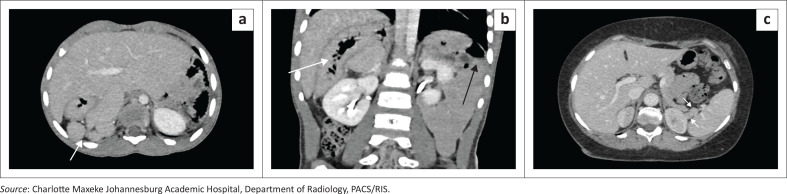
Heterotaxy syndrome identified incidentally in a 6-year-old trauma patient. (a, b) Axial and coronal contrast-enhanced CT images demonstrate multiple splenules (white arrow in a) in a patient with polysplenia associated with heterotaxy syndrome, with an empty splenic fossa (black arrow) and a right-sided stomach (white arrow in b). (c) Axial contrast-enhanced CT in a different patient demonstrates multiple small splenules adjacent to a dominant spleen (arrows), representing a normal anatomical variant (splenunculi).
